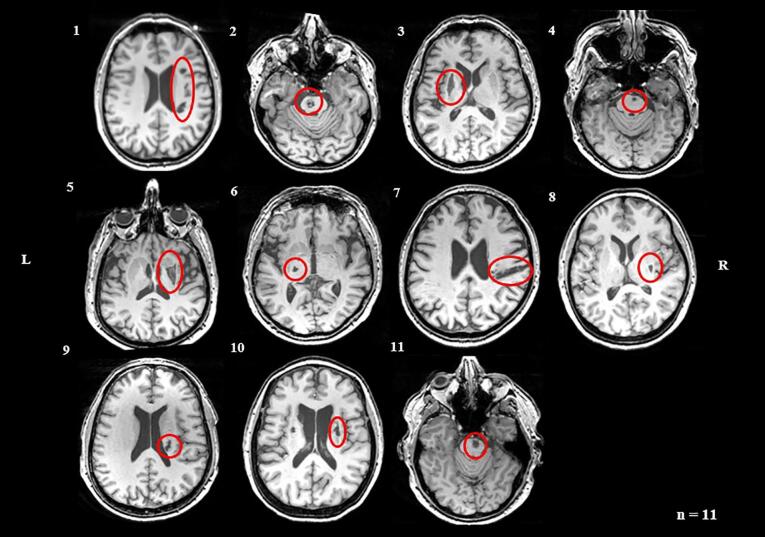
Axial structural T1-weighted anatomical scans at the level of maximum infarct volume for each stroke patient obtained at week 29 post-stroke. Arabic numbers denote the case numbers of patients. L = left; R = right.
